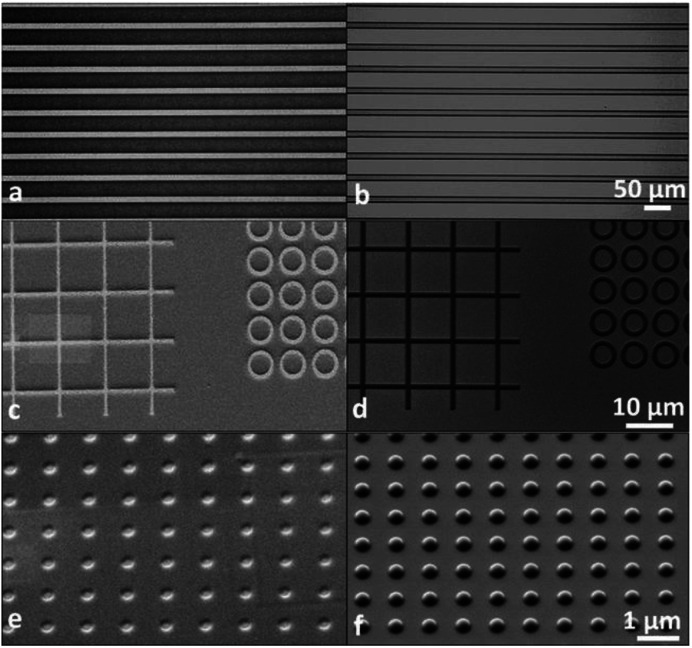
Optical and SEM images of the positive (a, c and e) and negative (b, d and f) developments obtained by UV (a and b), X-rays (c and d) and EB (e and f) lithography. Reprint with permission from ref. 35. Copyright 2013, Wiley-VCH Verlag GmbH &Co. KGaA.
